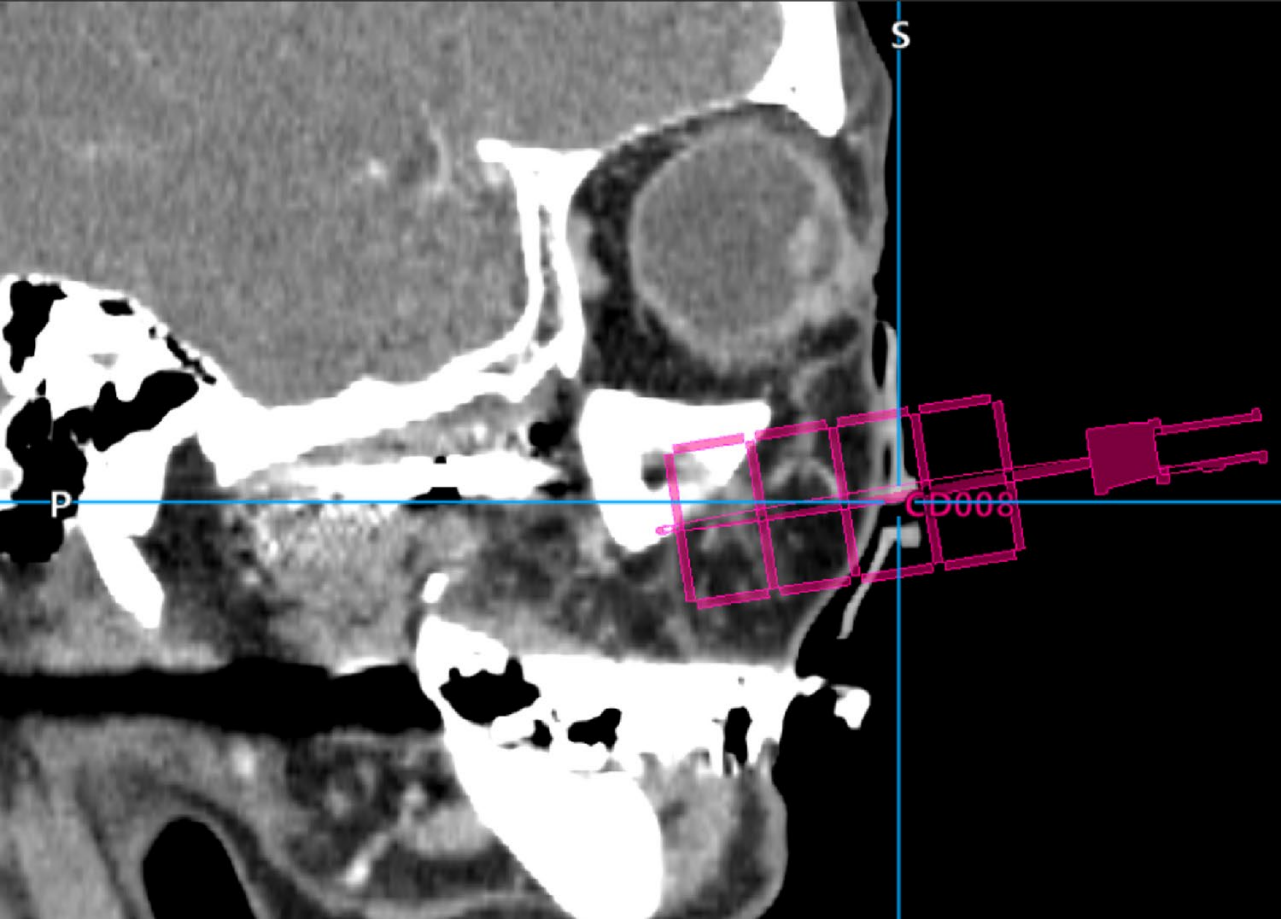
A sagittal reconstructed CT navigation image for NIR-PIT needle insertion. (Courtesy of Prof. Takayoshi Suzuki, Hokkaido University, Graduate School of Medicine)
